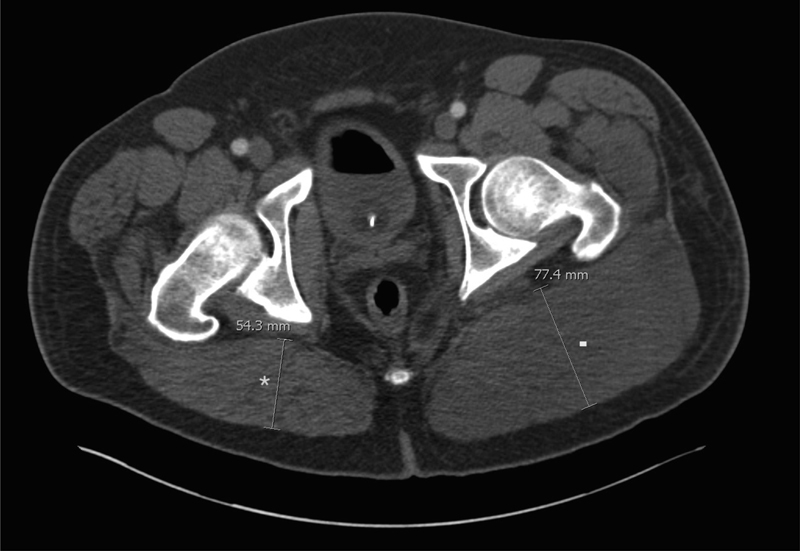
Computed tomography scan prefasciotomy showing net asymmetry of the gluteal muscles, especially the large left buttock measuring 77.4 mm (▪) versus 54.3 mm on the contralateral side (*).
